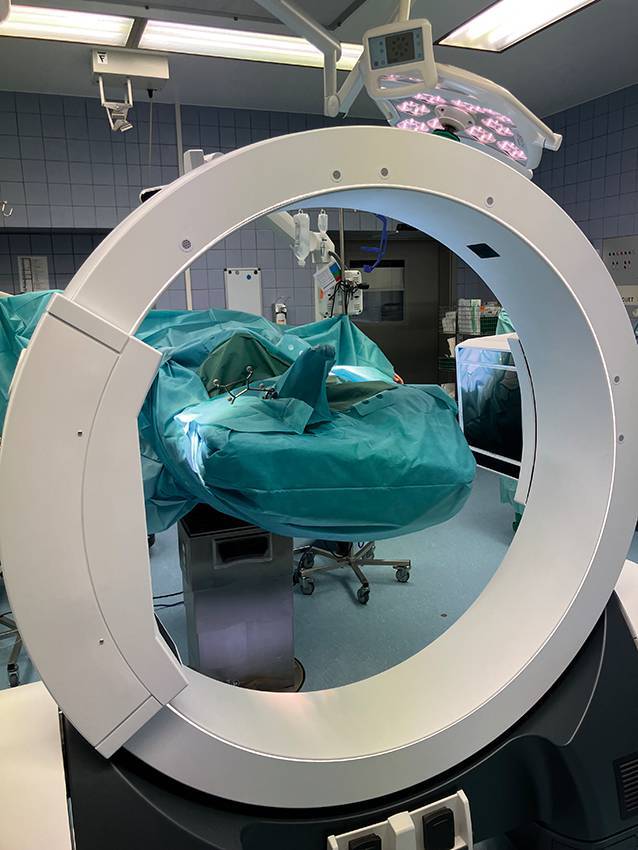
Das Cone-Beam-CT (CBCT) in Scan-Position
